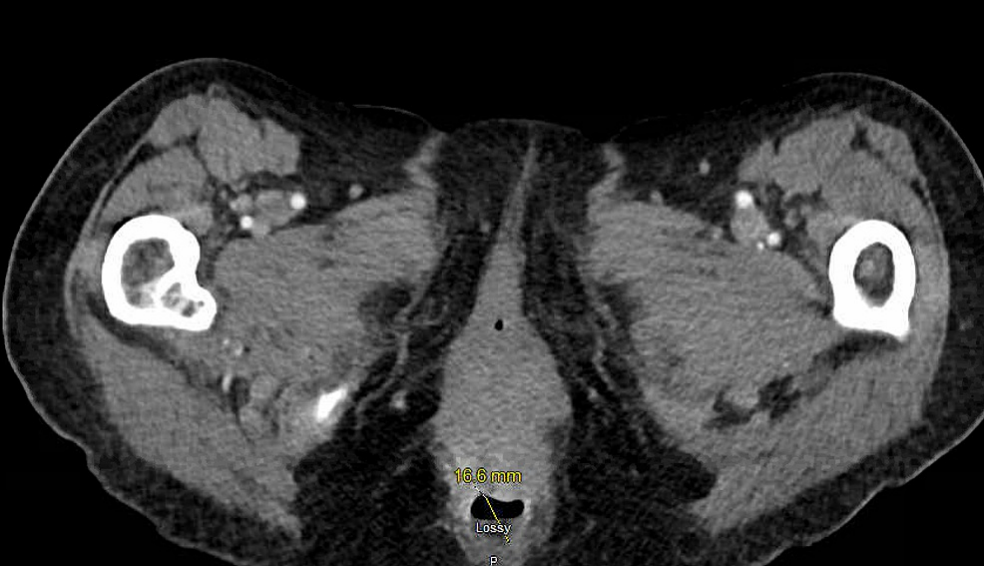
Perianal abscess identified on CT A/P
